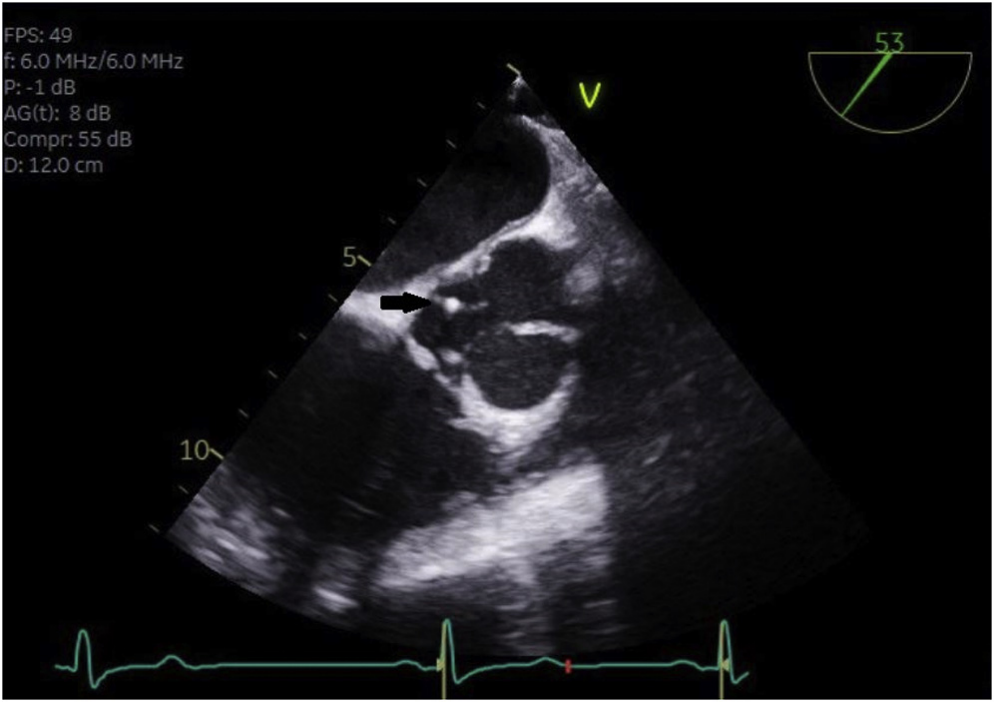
Transesophageal echocardiography: Wire crossing the noncoronary cusp.
